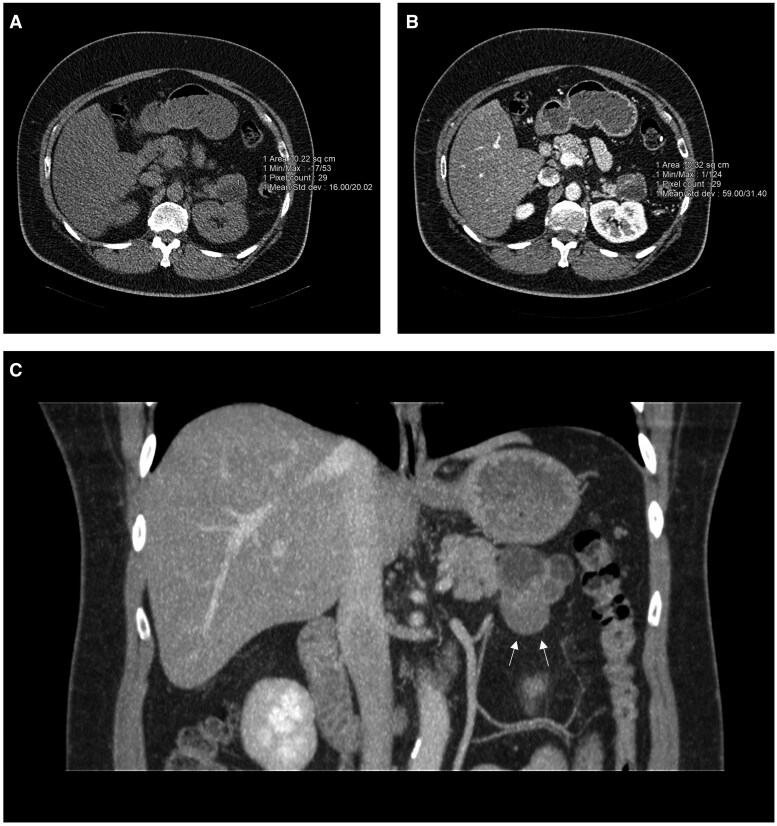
Computed tomography images showing a lobulated septated cystic mass in the tail of the pancreas. (A) Axial non-contrast and (B) arterial phase sequences demonstrating enhancement of the anterior solid component. (C) Coronal view of the mass in arterial phase (white arrows).
